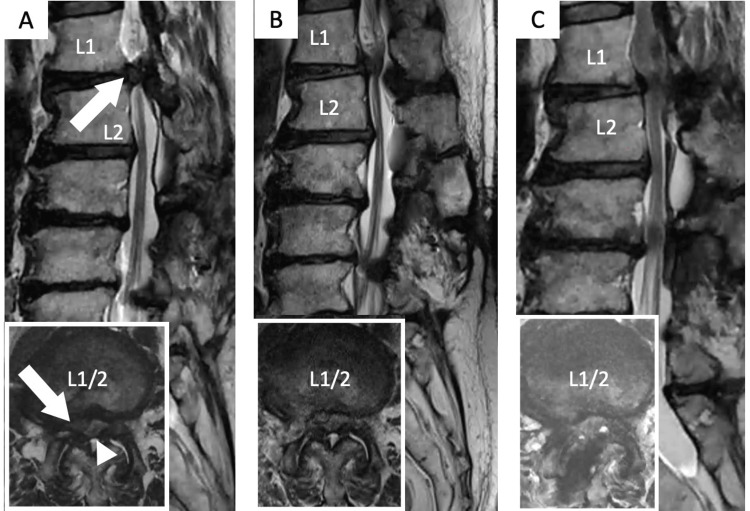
Case 2: A 75-year-old male with intermittent claudication and lower-extremity pain who underwent surgery twice. (A) Preoperative MRI revealing a posterolateral type disc herniation (arrow) with spinal canal stenosis (arrowhead) compressing the dural sac at L1–L2. (B) MRI after full endoscopic lumbar discectomy from the right side via transforaminal approach. (C) MRI after additional full endoscopic lumbar laminectomy from the right side via interlaminar approach (This case is original and has not been previously reported.)
